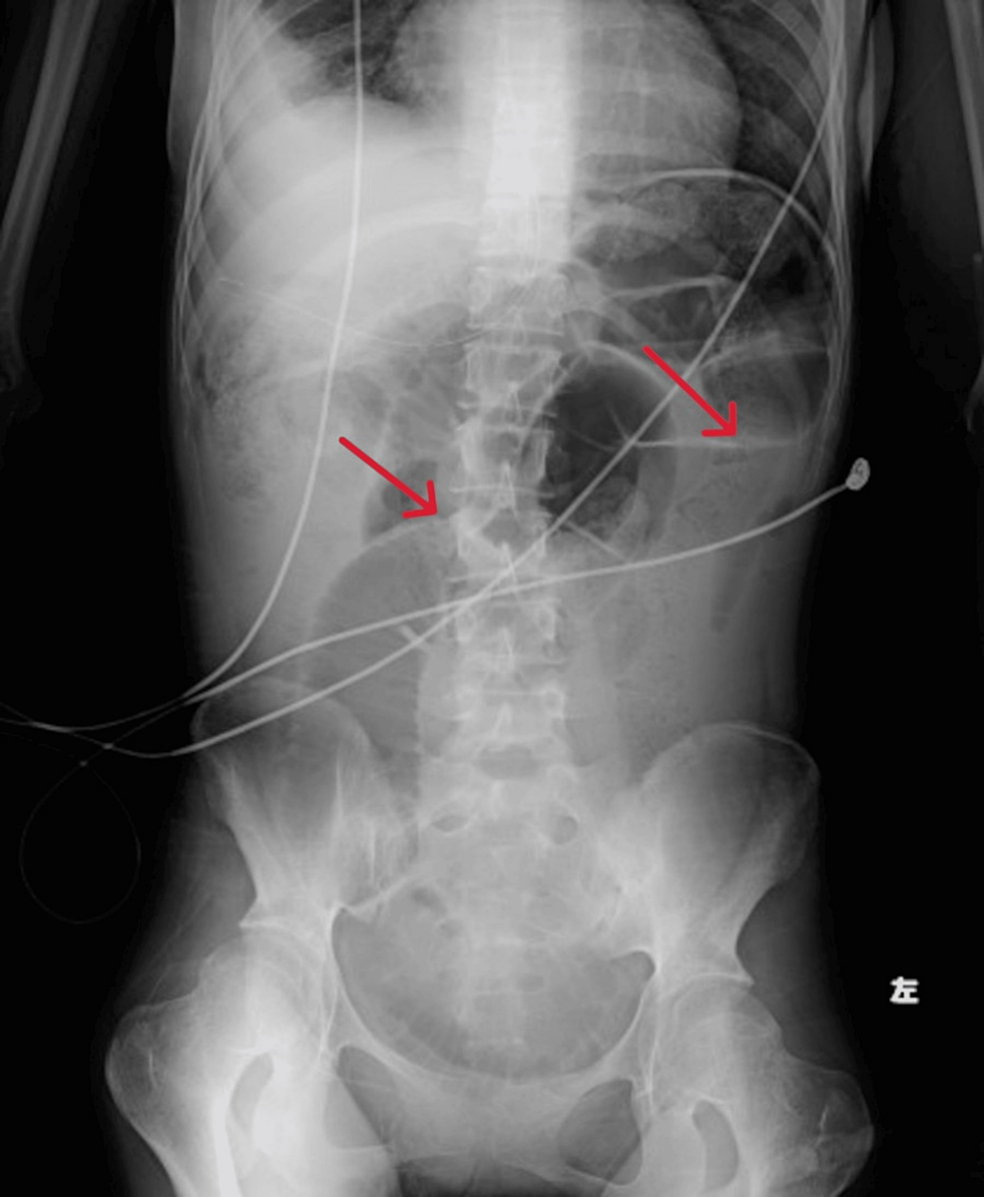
On day 31 after admission she had frequent vomiting and an abdominal X-ray showed marked dilatation of the intestinal tract.
